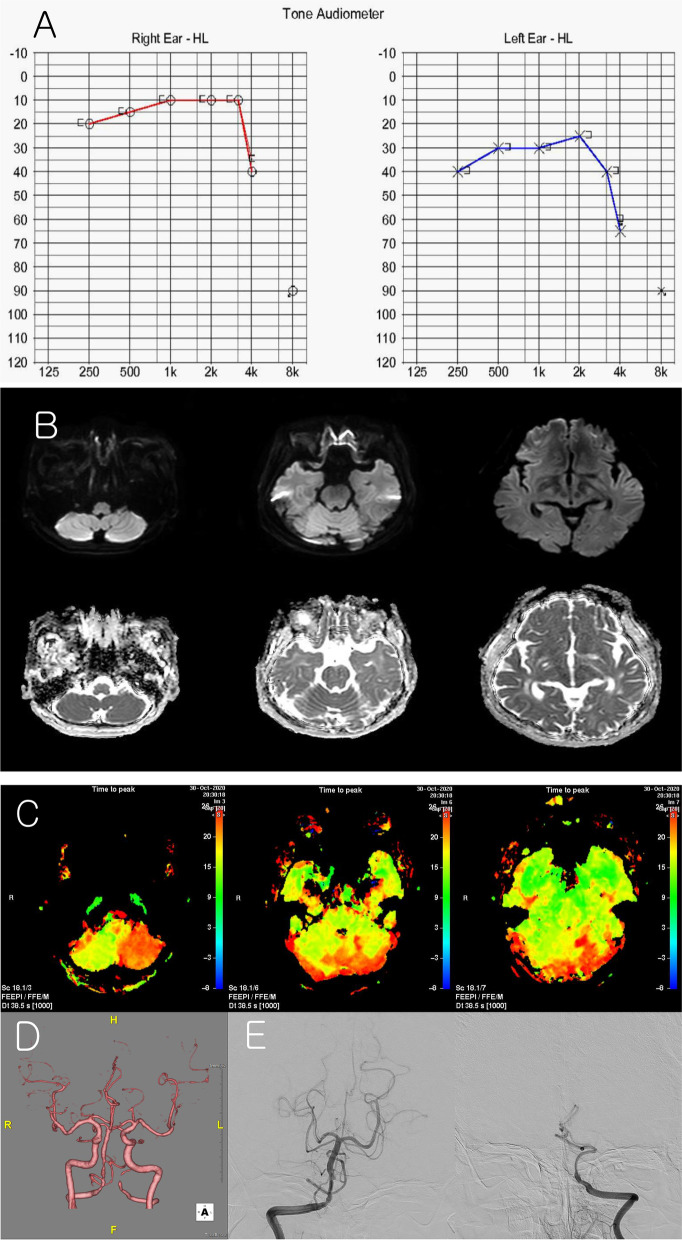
Pure-tone audiogram (a) showed a mild unilateral sensorineural, pure-tone average of 37 dB (dB) in the left ear. Diffusion-weighted imaging and apparent diffusion coefficient map (b) showed normal findings. Perfusion-weighted imaging (c) revealed left PICA and AICA territorial perfusion deficits without infarction. Brain CT angiography (d) revealed occlusion of the left post-PICA intracranial VA and multifocal stenosis of the right intracranial VA, indicating that both VA dissections were confirmed by conventional angiography (e)
